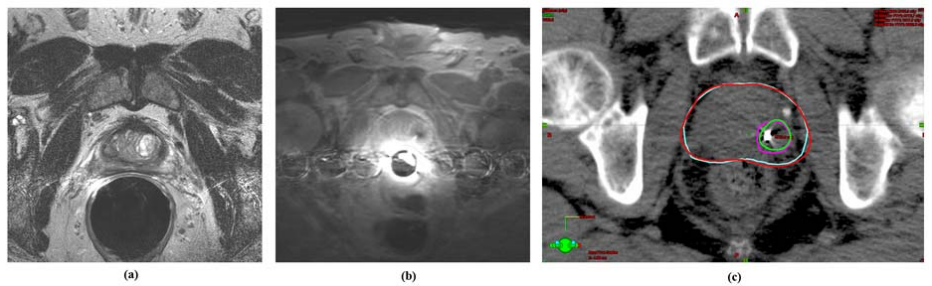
1a: Dynamic Contrast Enhanced (DCE) MR image showing region of increased gadolinium uptake in the left peripheral zone. 1b: MRI guided biopsy showing needle in the same region as in frame a. Pathology showed Gleason Score 7 disease. Immediately afterward, a fiducial marker was also placed in this location. 1c: Treatment planning image showing a fiducial marker in same region as figure a and b. The target was defined by fusing a treatment planning MRI (not shown and without an endorectal coil in place) with the treatment planning CT. The isodose lines are shown on the CT where the fiducial marker is best seen. The planning target volume of the intra-prostatic lesion is shown in fuschia. The 94.5 Gy isodose line is shown in green. The planning target volume of the prostate is shown in blue. The 75.6 Gy isodose line is shown in red.
